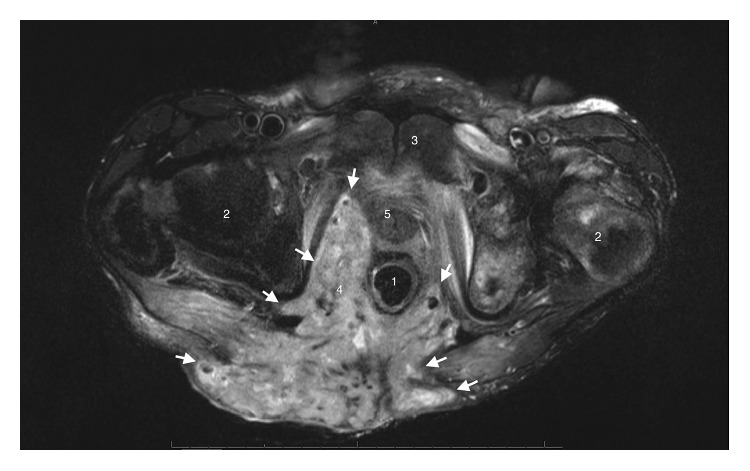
MRI of the pelvis shows extensive infiltration of the mass (indicated by solid arrow) into pelvic structures. (1) Rectum, (2) femoral head, (3) symphysis pubis, (4) ischiorectal fossa, and (5) urethra.
